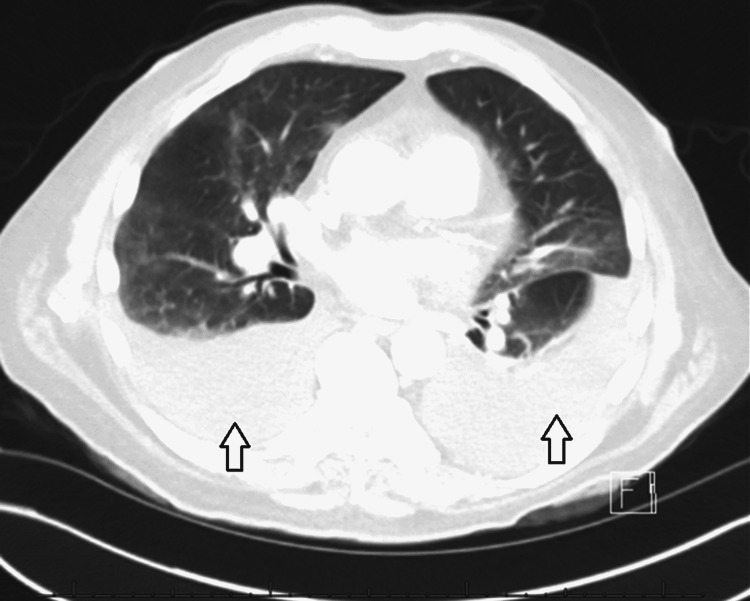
Pre-thoracentesis chest CT showing moderate bilateral opacities (arrows) with mild patchy opacity and bronchiectasis in the right upper lobe, potentially scarring or fibrosis.
